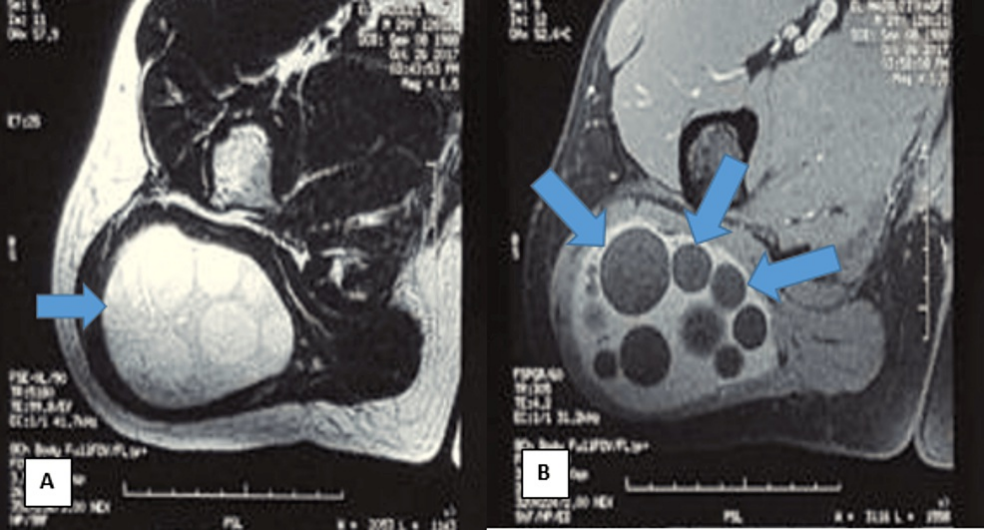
MRI of the pelvis reveals a cystic formation A: Axial T1-weighted imaging displays a cyst infiltrating the right gluteus maximus muscle (blue arrow). B: Axial T2-weighted imaging unveils the appearance of a multivesicular cyst infiltrating the right gluteus maximus muscle (three blue arrows).
